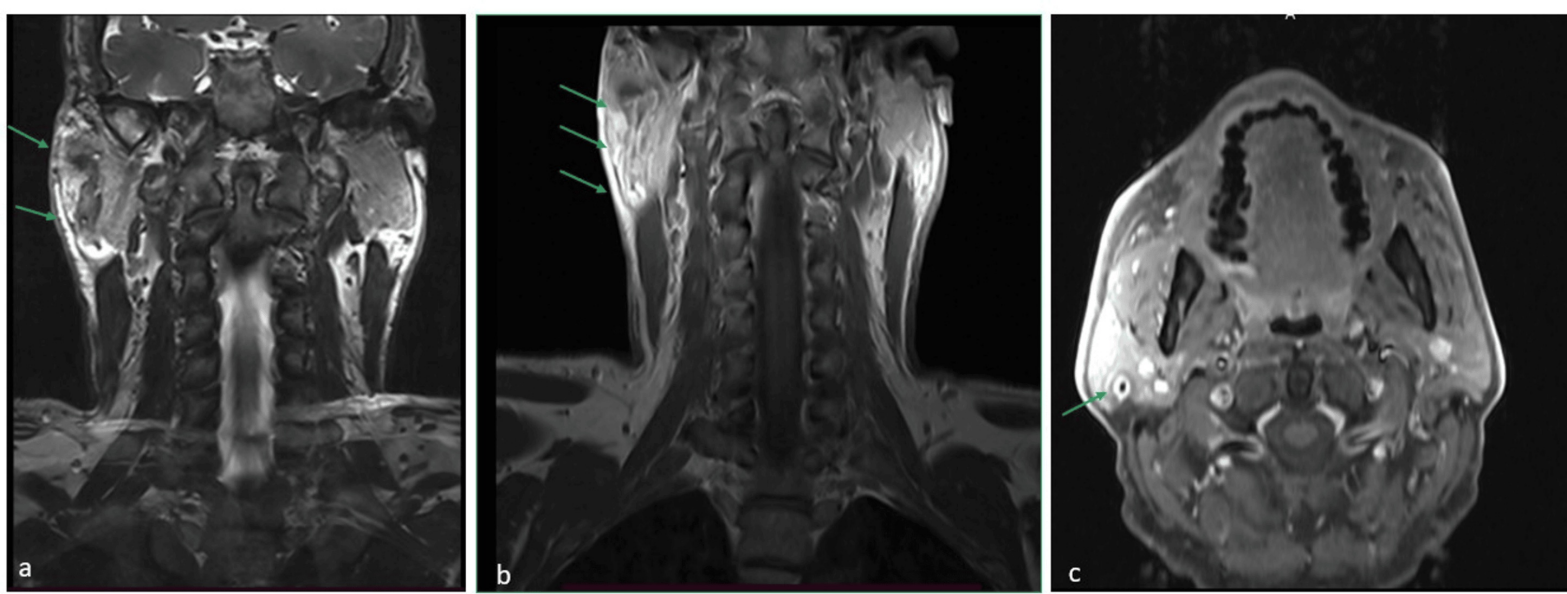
MRI showed an intraparotid lesion in the superficial layer, resembling a fistula (green arrows). The fistula tract varied in diameter, with the widest part measuring 1.6 cm in the middle. The external auditory canal and right tympanic membrane appeared normal, indicating no involvement of these structures. Nearby ear structures were not affected. (a) T2 sequence, coronal view; (b) T1 sequence, coronal view; (c) T1 sequence, axial view
